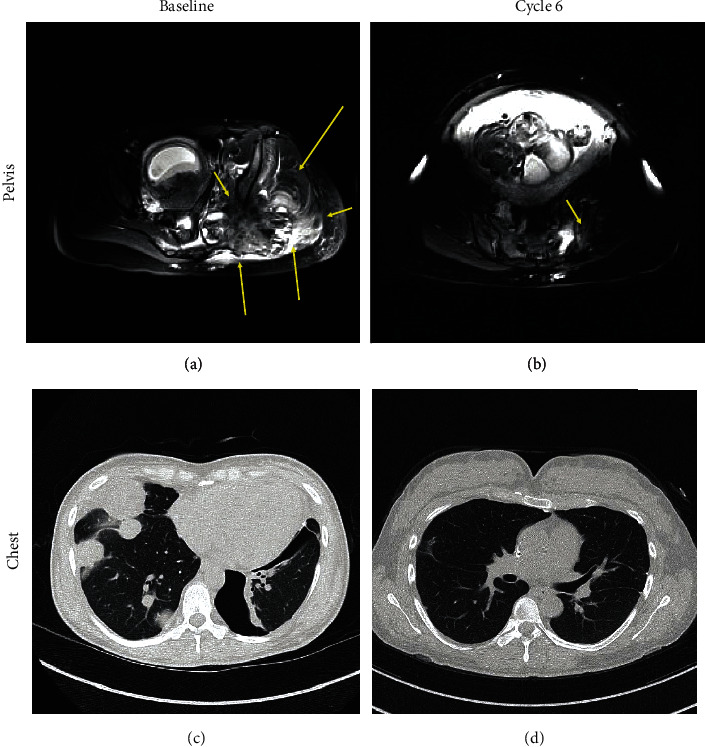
Computed tomography scans of (a, b) pelvis and (c, d) chest for case 3. CT scan was performed at baseline prior to starting treatment (a, c) and again after 6 cycles and 4.5 months of therapy (b, d). Resolution of lung metastases and pneumothorax is observed.
